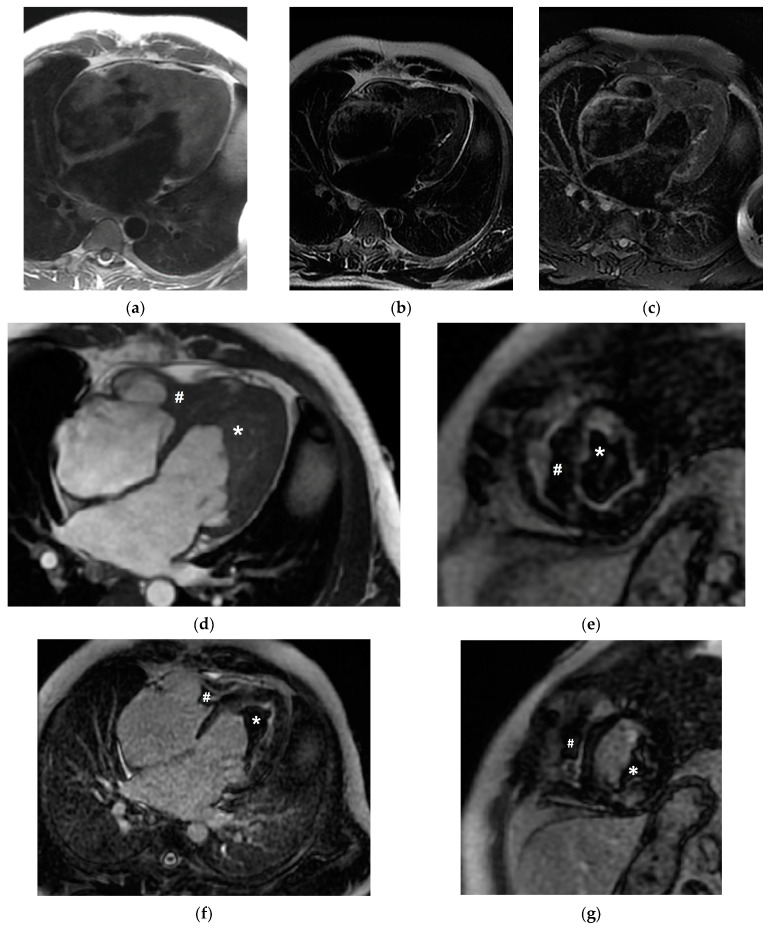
CMR images revealing left (*) and right (#) intraventricular masses. (a) T1-weighted 4C view. (b) T2-weighted 4C view. (c) T2-weighted fat-suppressed 4C view. (d) 4C-balanced free steady-state precession 4C view. (e,g) Late gadolinium-enhanced SA view. (f) Late gadolinium-enhanced 4C view.
